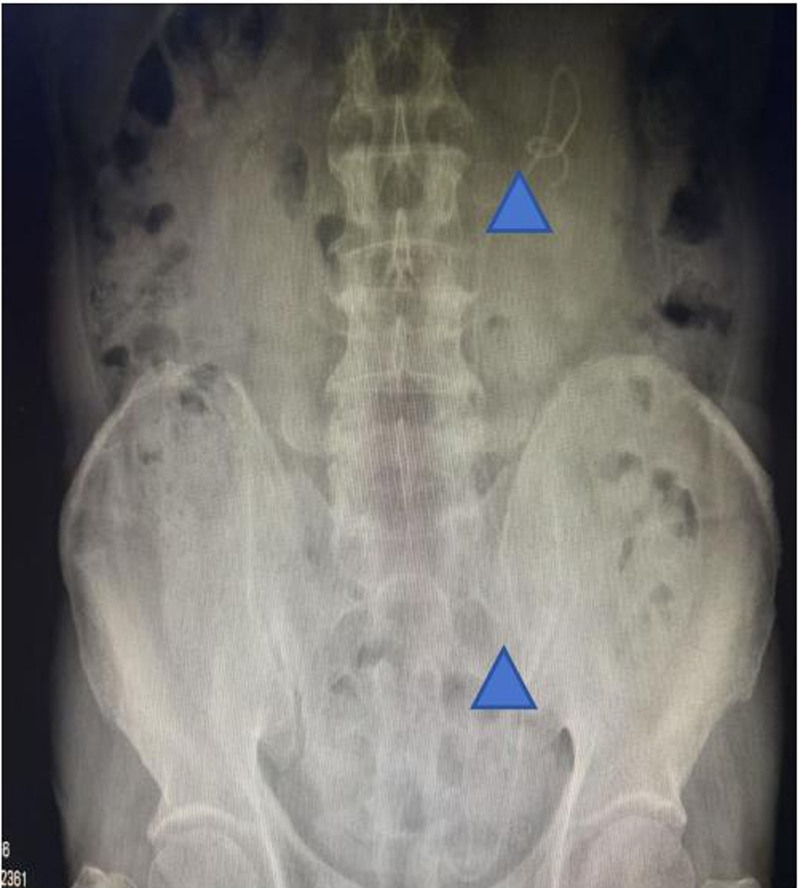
Abdominal radiograph, ureteral stent position (indicated by triangular star).
